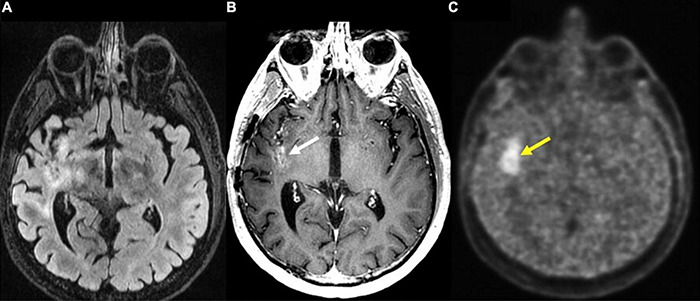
Recurrent glioblastoma in right posterior insula (FMISO PET-MR). (A) Axial FLAIR: Ill-defined hyperintense area in the right posterior insula (black arrow); (B) axial T1 post-contrast: mild enhancement in the right posterior insula (white arrow); (C) axial ^18^F-FMISO PET: avid uptake of FMISO tracer in the right posterior insular (yellow arrow). Biopsy targeted to this region showed recurrent glioblastoma. ^18^F-FMISO, Fluoromisonidazole; PET, Positron emission tomography.
